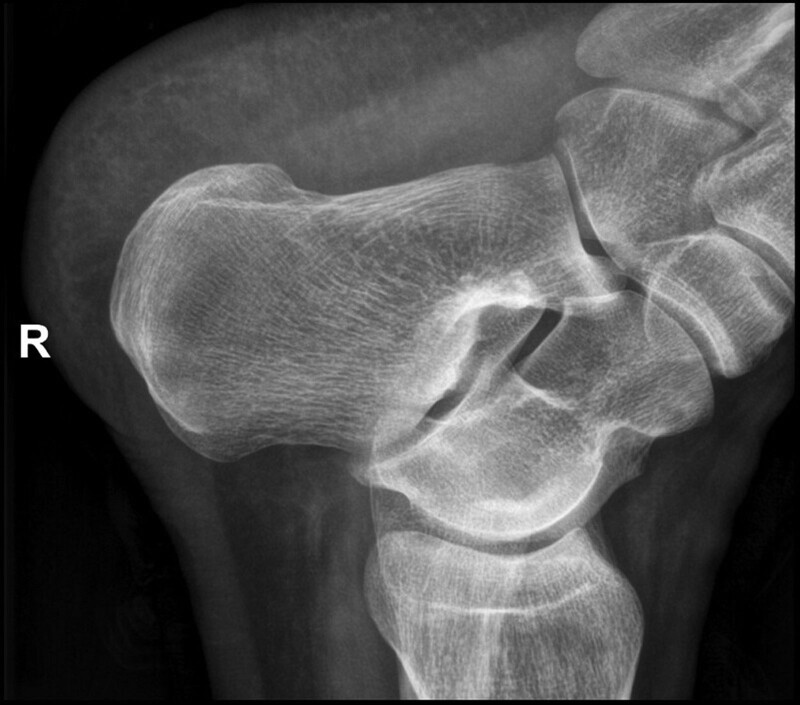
Right heel X-ray showing the intact bone structure of the right heel without obvious bone abnormalities.
